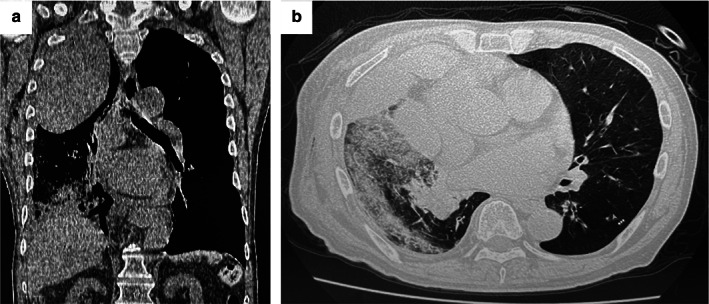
(a) Emergent chest computed tomography on 15 POD showed a huge extrapleural hematoma on the right anterior chest wall ranged to thoracic apex and (b) widespread ground glass opacification in the right lower lobe
